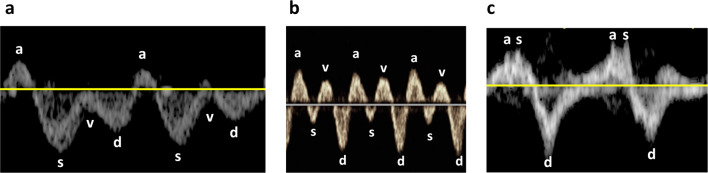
a Normal HV Doppler waveforms exhibit a small retrograde a-wave, followed by anterograde S and D waves, with the S wave being deeper than the D wave. b In mildly abnormal HV waveforms, the S wave is less deep than the D wave. c Severely abnormal HV waveforms show a reversal of the S wave, with only the D wave as a negative deflection wave; *HV* Hepatic Vein
